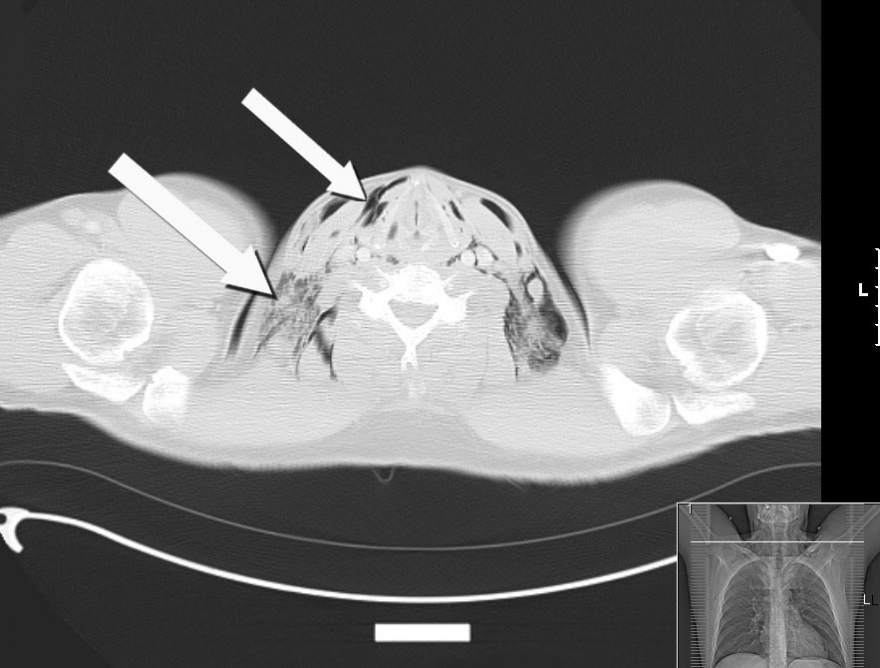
Axial section CT neck/thorax showing subcutaneous emphysema and pneumomediastinum.
